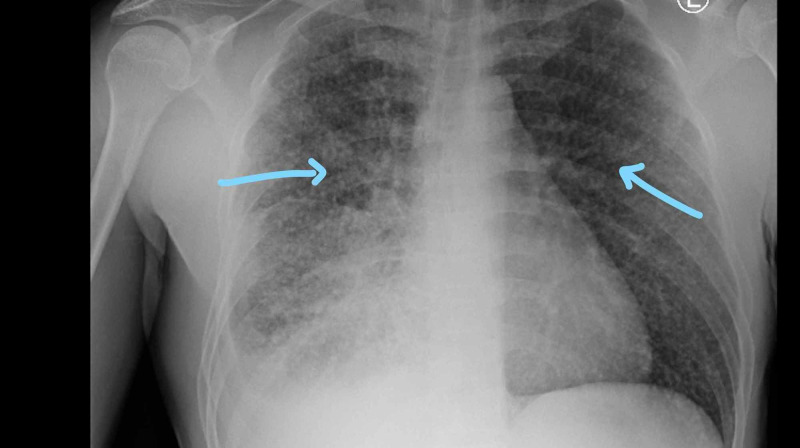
Chest x-ray showing diffuse bilateral opacities Arrows pointing towards diffuse bilateral opacities
